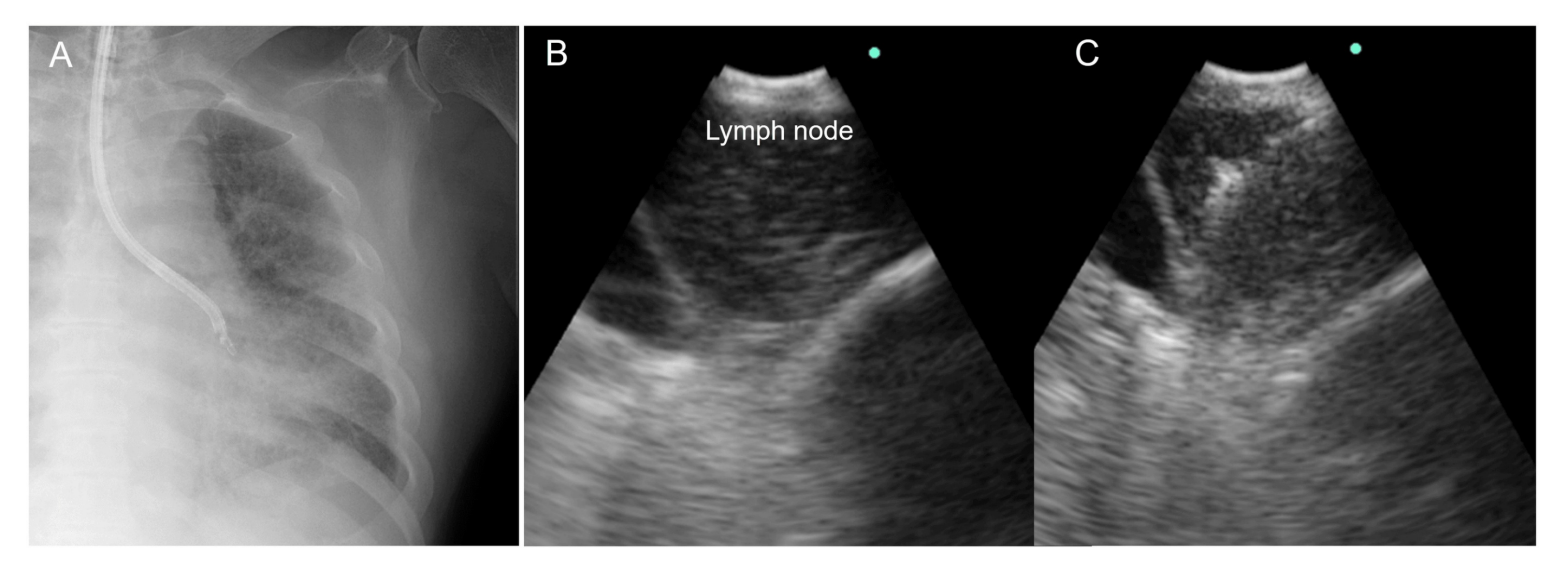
Endobronchial ultrasound images. (A) X-ray shows a convex probe adjacent to the target lesion. (B) An endobronchial ultrasound (EBUS) image confirming an enlarged #12 lymph node. (C) The mini-forceps were inserted into the left #12 lymph node, and an opening in the lymph node was confirmed.
